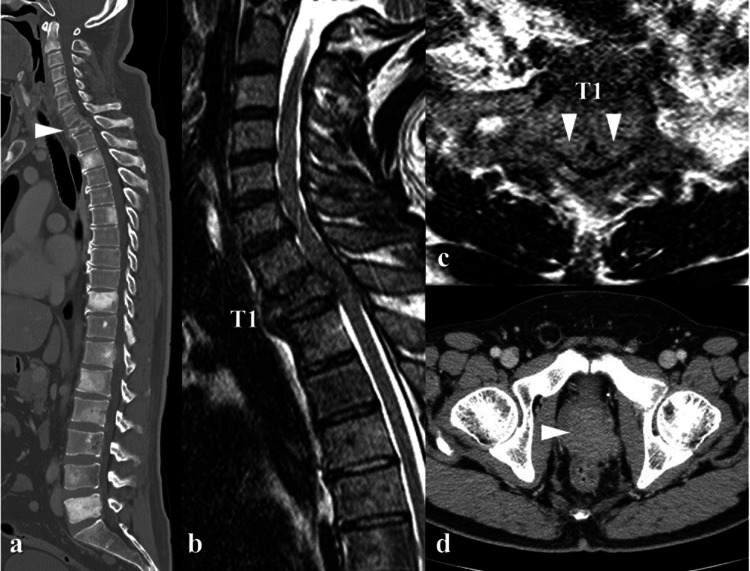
Preoperative images. (a) Whole spinal CT showing multiple osteoblastic lesions and pathologically collapsed T1 vertebral body (arrowhead). (b, c) T2-weighted MRI images showing severe spinal cord compression due to an epidural lesion (arrowheads). (d) CT showing an enlarged prostate (arrowhead).
